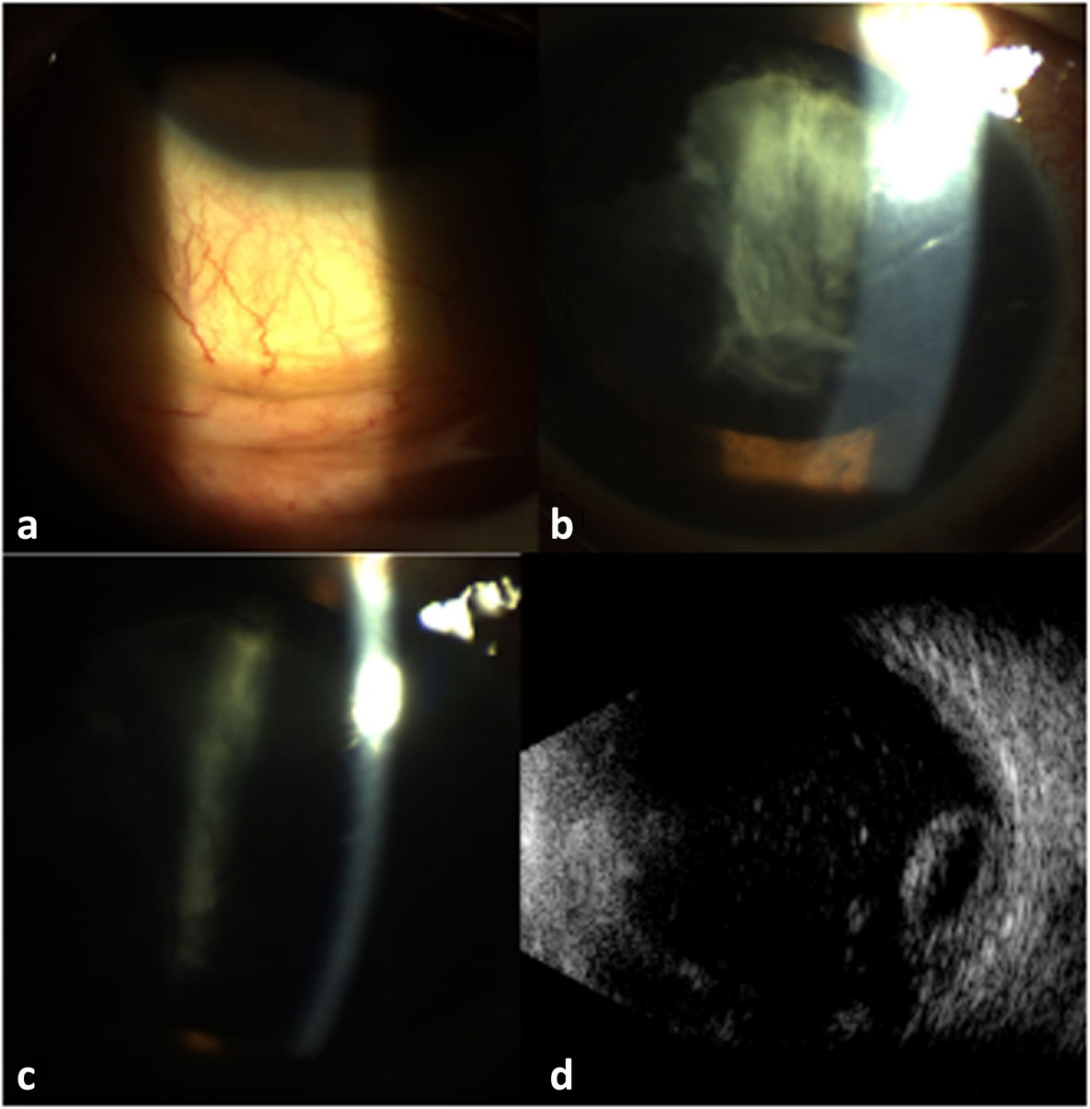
(a) Slit-lamp photography seven days after pars plana vitrectomy showing a mild conjunctival hyperemia and hypopyon. (b,c) Three days after initiation of topical steroid therapy, the hypopyon has resolved. Note the lack of typical lens capsule convexity and the residual cortical material adherent to the anterior lens capsule. (d) B-scan ultrasonography shows a globular echogenic structure in the inferior posterior vitreous cavity resting on the retina consistent with a dislocated lens nucleus
